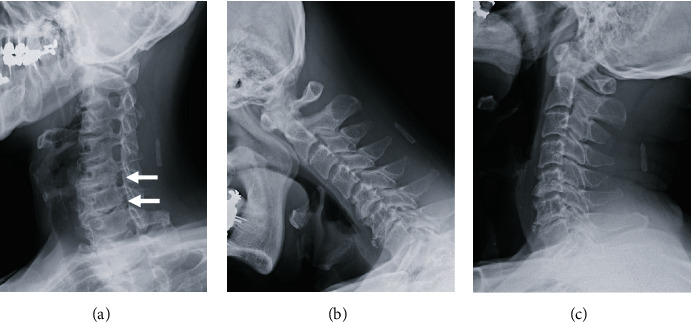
Cervical spine X-ray images. The left oblique view (a) revealed the left C5-6 and C6-7 stenoses (arrow). Anteflexion (b) and retroflexion (c) did not show cervical spine instability.
